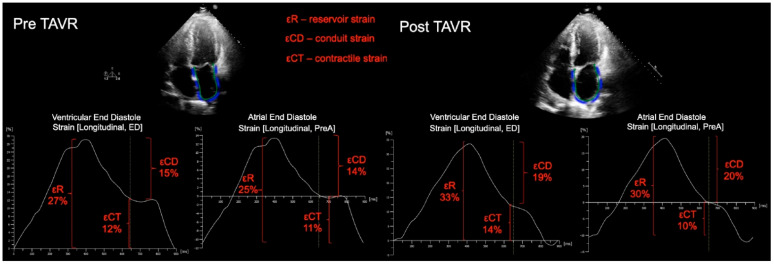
Representative example of LA strain measured for a patient prior to, and following TAVR, showing improvement in LA phasic function. For LA strain analysis, apical 4-chamber and 2-chamber views were used (4-chamber views only shown); the LA endocardial border was manually traced, generating a region of interest (ROI). Following segmental tracking and manual adjustment of the ROI, the software package generated longitudinal strain curves. LA strain was measured with the zero-reference standard at end-diastole and at the onset of atrial contraction. Reservoir function (εR), (strain value at mitral valve opening—ventricular end-diastole), LA conduit function (strain at onset of atrial contraction—strain at mitral valve opening) and contractile function (εCT) (strain at ventricular end-diastole—strain at onset of atrial contraction) were then derived.
